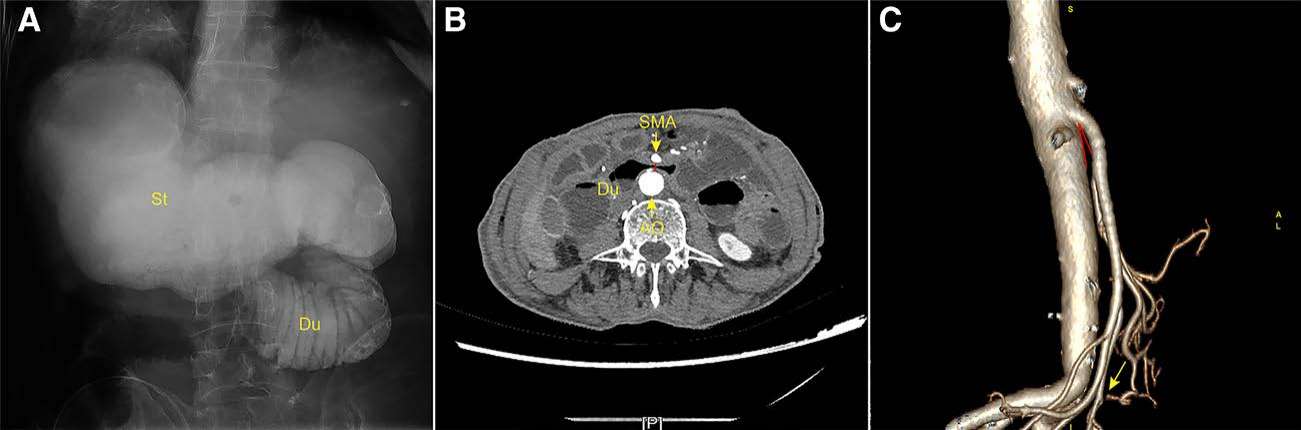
(A) Upper GI series showed a dilated stomach and proximal duodenum, a straight-line cutoff at the third part of the duodenum; (B) The distance between the aorta and SMA on CT was 4 mm; (C) The angle between the aorta and SMA on CT was 20°. Cecal-rectal anastomosis causes relative stretching of the SMA and flattens it against the aorta. Ao = aorta, Du = duodenum, SMA = superior mesenteric artery, St = stomach.
